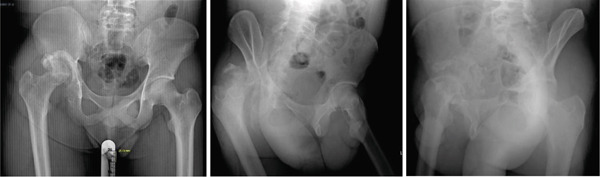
Case 1 preoperative radiographs.
